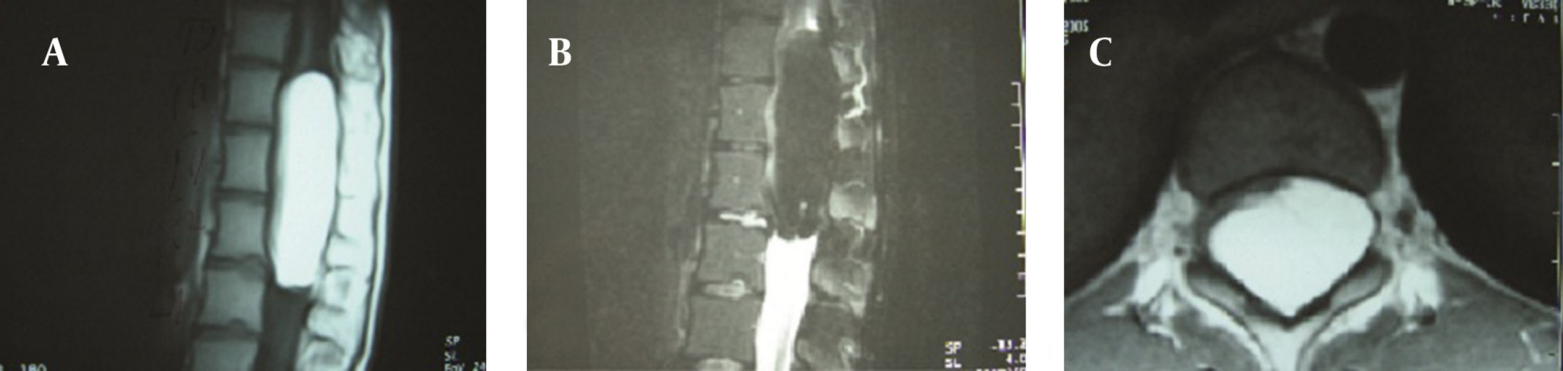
MR Images of Case 2. A pre-contrast T1-weighed sagittal MRI, showing a high signal intensity tumor from Th10 to L1 level (A). The signal intensity dropped dramatically with fat saturation technique, confirming fat as its main component (B). Axial T1W image demonstrates the mass located in an intramedullary position (C).
